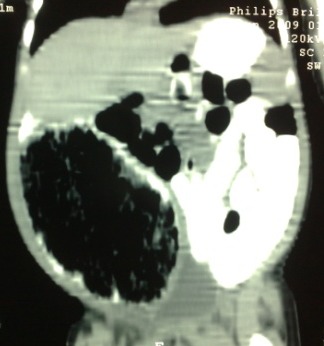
Figure 3: CT scan of the abdomen showing huge cyst in the right abdomen
